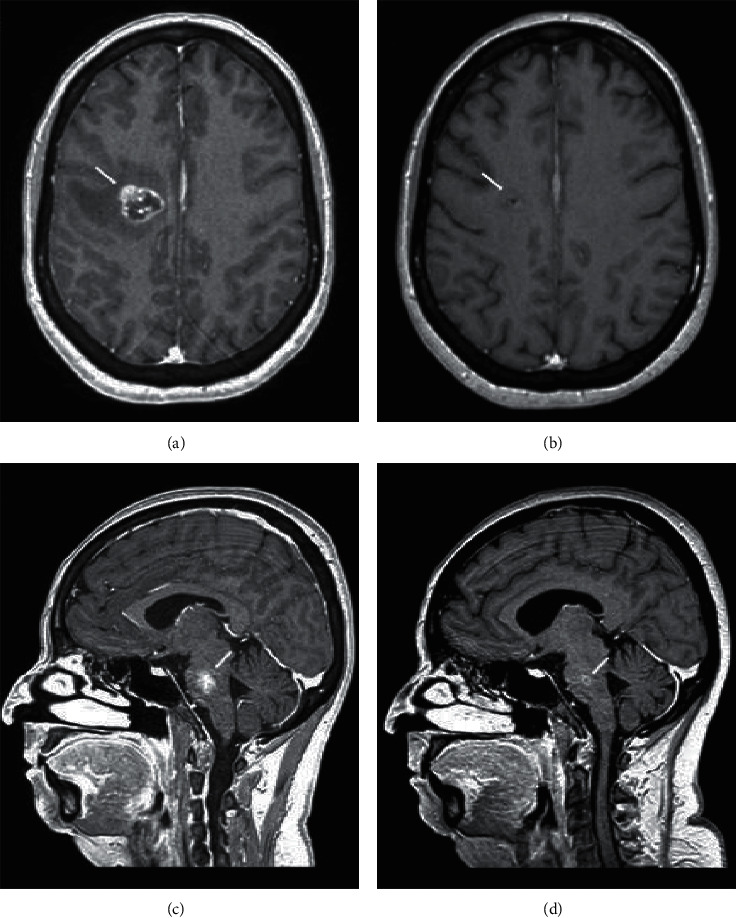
Dynamics of brain metastases on osimertinib. Brain MRI (T1 with contrast, axial, and sagittal) showing brain metastases (white arrows) before treatment (a and c) and 1 month after initiating treatment (b and d).
